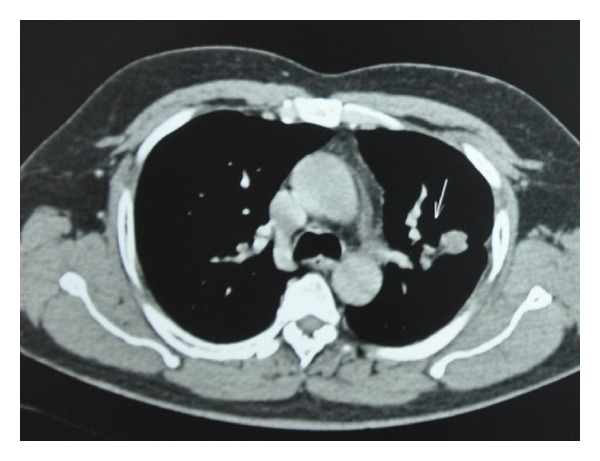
After 4 cycles of chemotherapy CT scan of the lung (transverse section) showing reduction in the size of the lung lesion; measuring 3.0∗2.1 cms in the left upper lobe.
